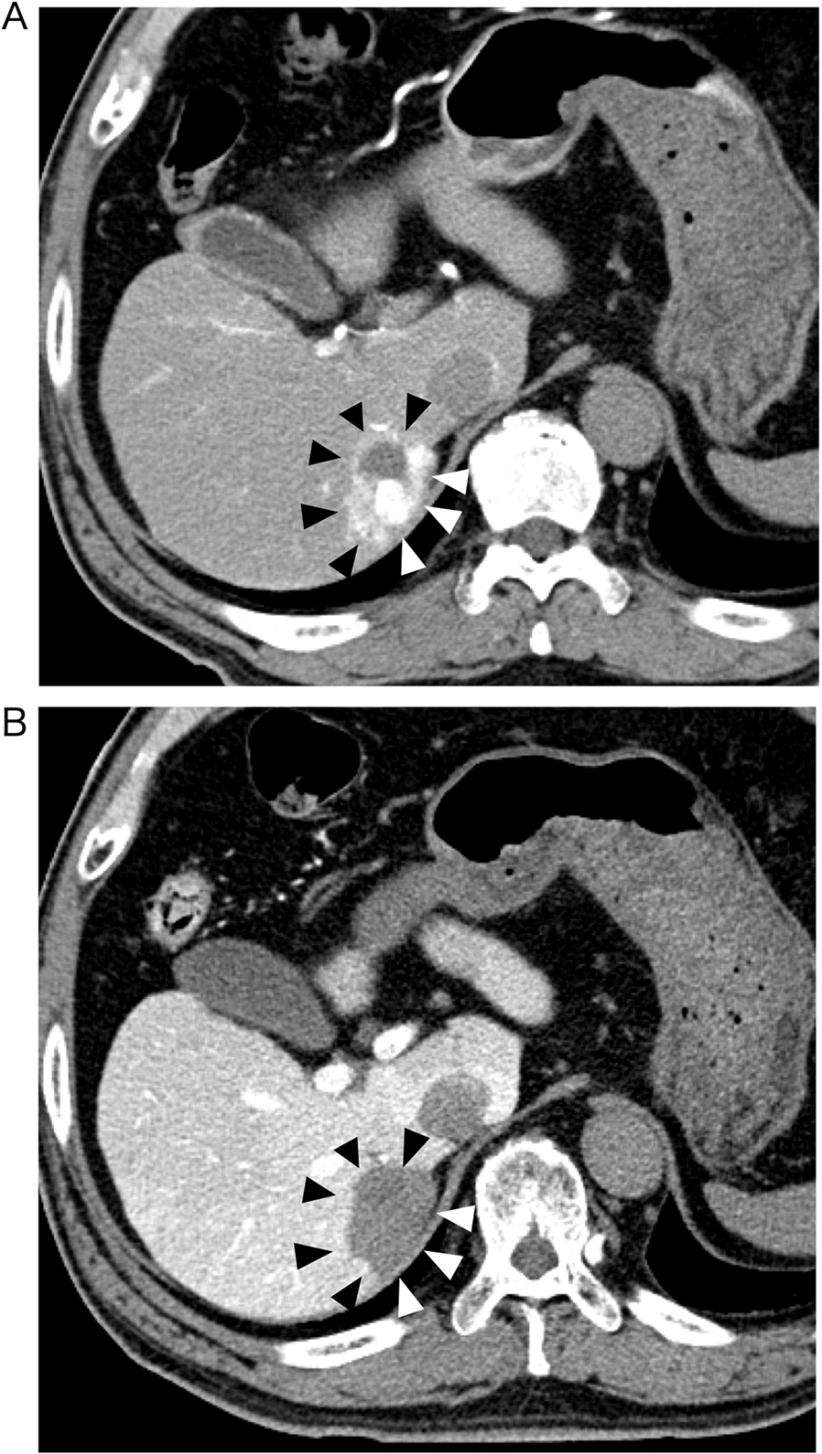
CT images with transcatheter angiography of the tumor. (Shown with arrow heads). A Arterial phase. The tumor shows early enhancement in a mosaic pattern. B Portal phase. Contrast is washed out in the portal phase
